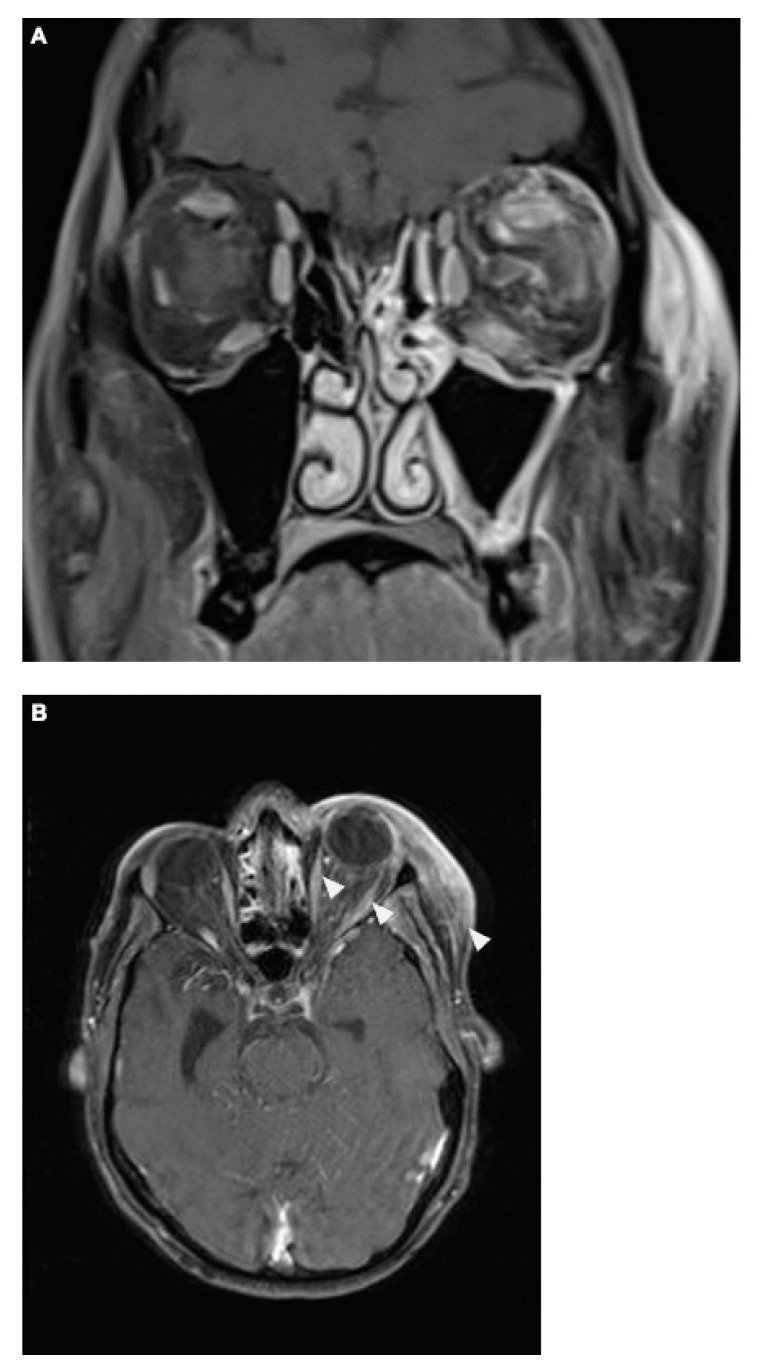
The coronal T1-weighted post-Gadolinium magnetic resonance imaging (MRI) sequence revealed no definitive evidence of cavernous sinus thrombosis. However, venous dilation in the left superior ophthalmic vein was noted, along with significant phlegmonous enhancement at the apex of the left orbit, involving the left superior rectus muscle and potentially the optic nerve. Adjacent sinusitis was observed in the left frontal, ethmoid, sphenoid, and maxillary sinuses, accompanied by mild swelling of the left eyelid and inflammation of the adjacent subcutaneous tissue (A). The axial T1 post-Gadolinium MRI sequence (B) demonstrated contrast-enhanced protrusion of the left eye muscles, particularly affecting the superior rectus muscle. This indicated ongoing inflammation, though there was a slight reduction in the extent of optic nerve involvement compared to earlier scans, suggesting a partial therapeutic response. Importantly, no signs of cavernous sinus thrombosis were observed (as described above), and the venous structures remained patent.
